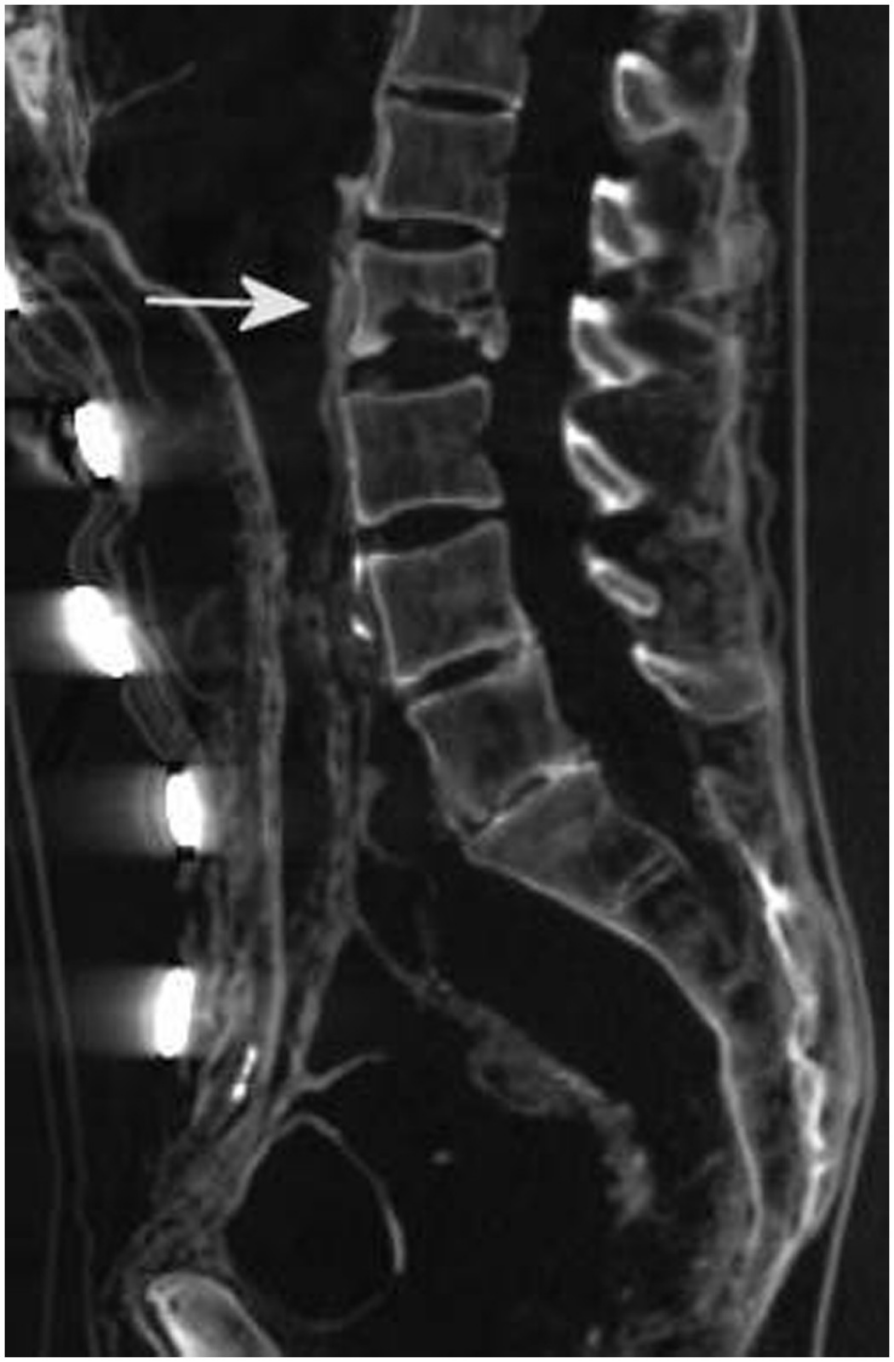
CT scan of the lumbar vertebra showing a localised irregularly-shaped osseous defect of L2 (arrow).
